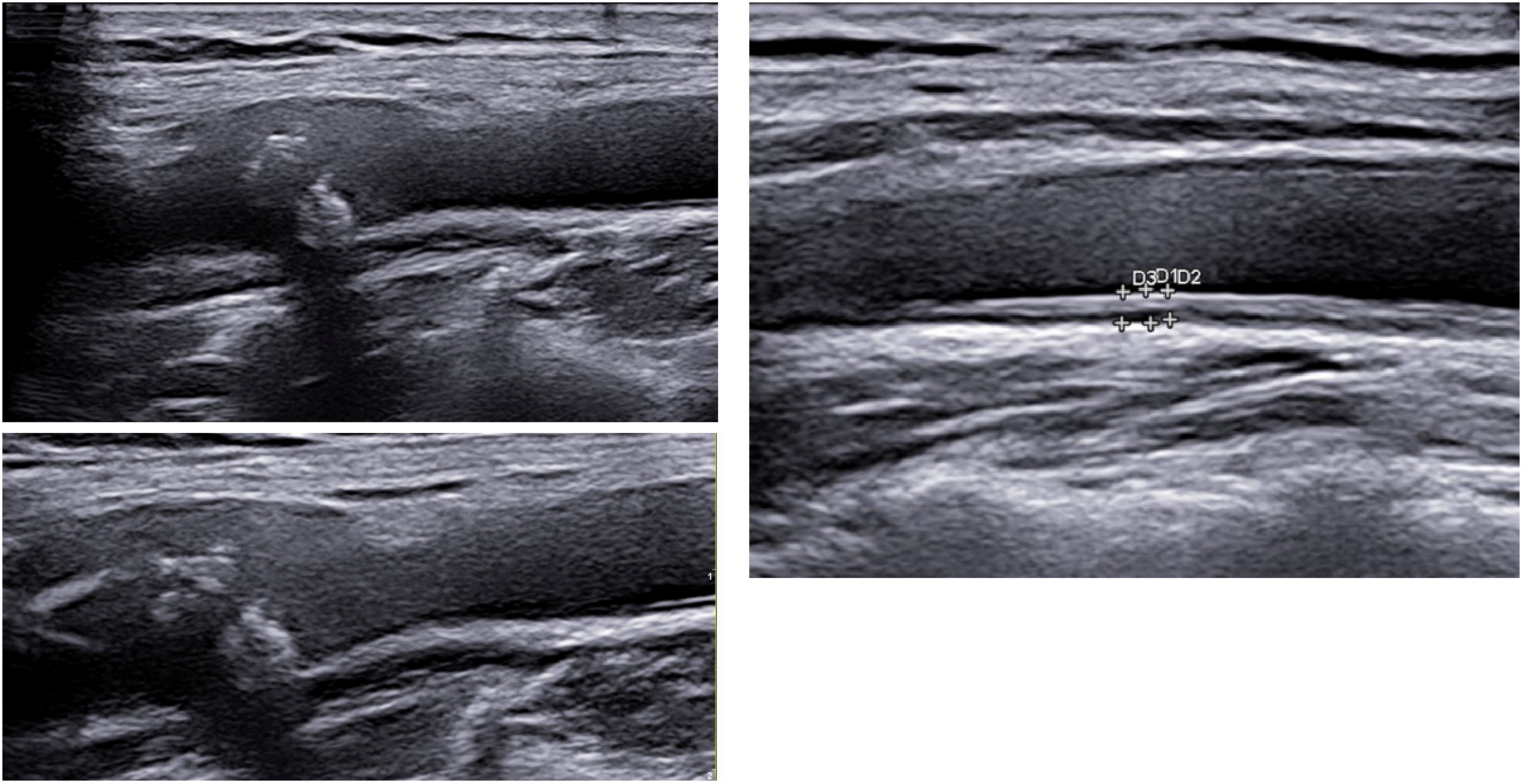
Carotid ultrasound imaging with plaque (left) and increased carotid intima media thickness (above) in two different patients, courtesy by Dr. Konstantinos Triantafyllias, Rheumatology center in Bad Kreuznach, Germany.
